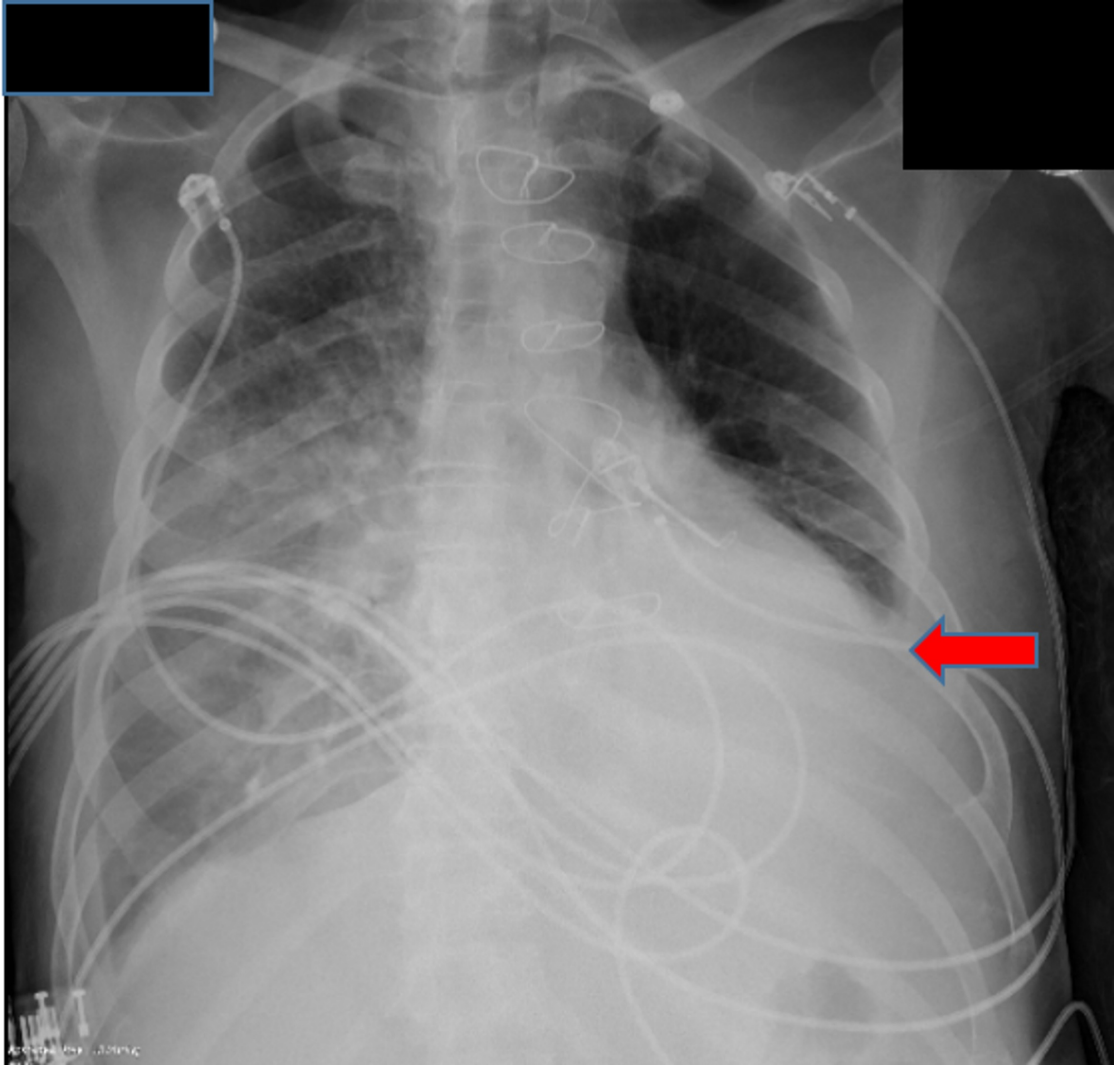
Subsequent chest X-ray showing small left sided pleural effusion (red arrow)
